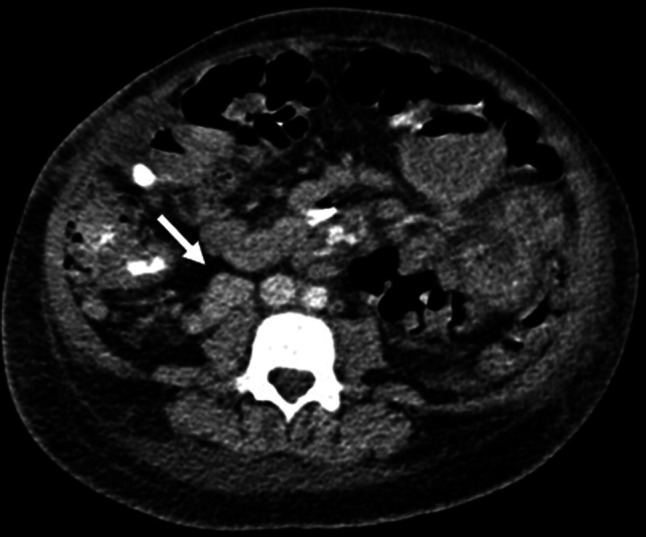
Contrast-enhanced computed tomography scan of a 10-year-old girl after liver transplant showed a 1.7 × 3.3 × 1.5 cm right retroperitoneal lymph node.
